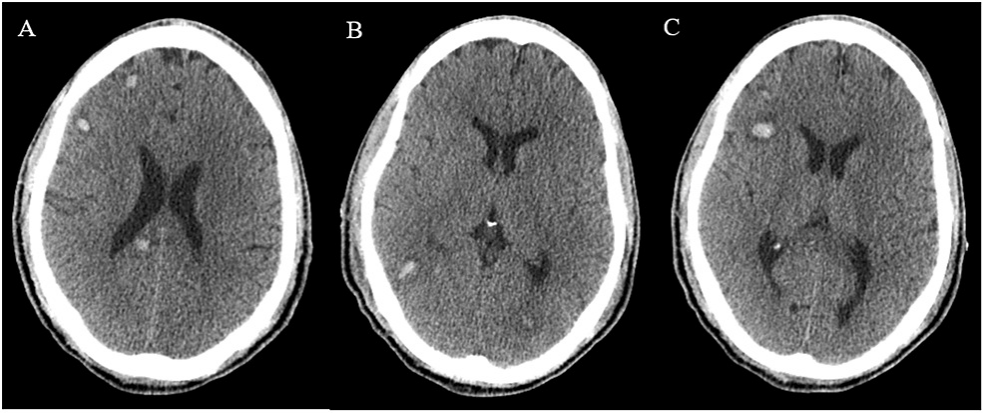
Computer tomography (CT) brain. Multiple foci of acute parenchymal hemorrhage involving both cerebral hemispheres mostly at the gray-white junction, with associated surrounding edema (A, B). The majority of the lesions measure under 1 cm with the dominant lesion in the right frontal lobe (C).
